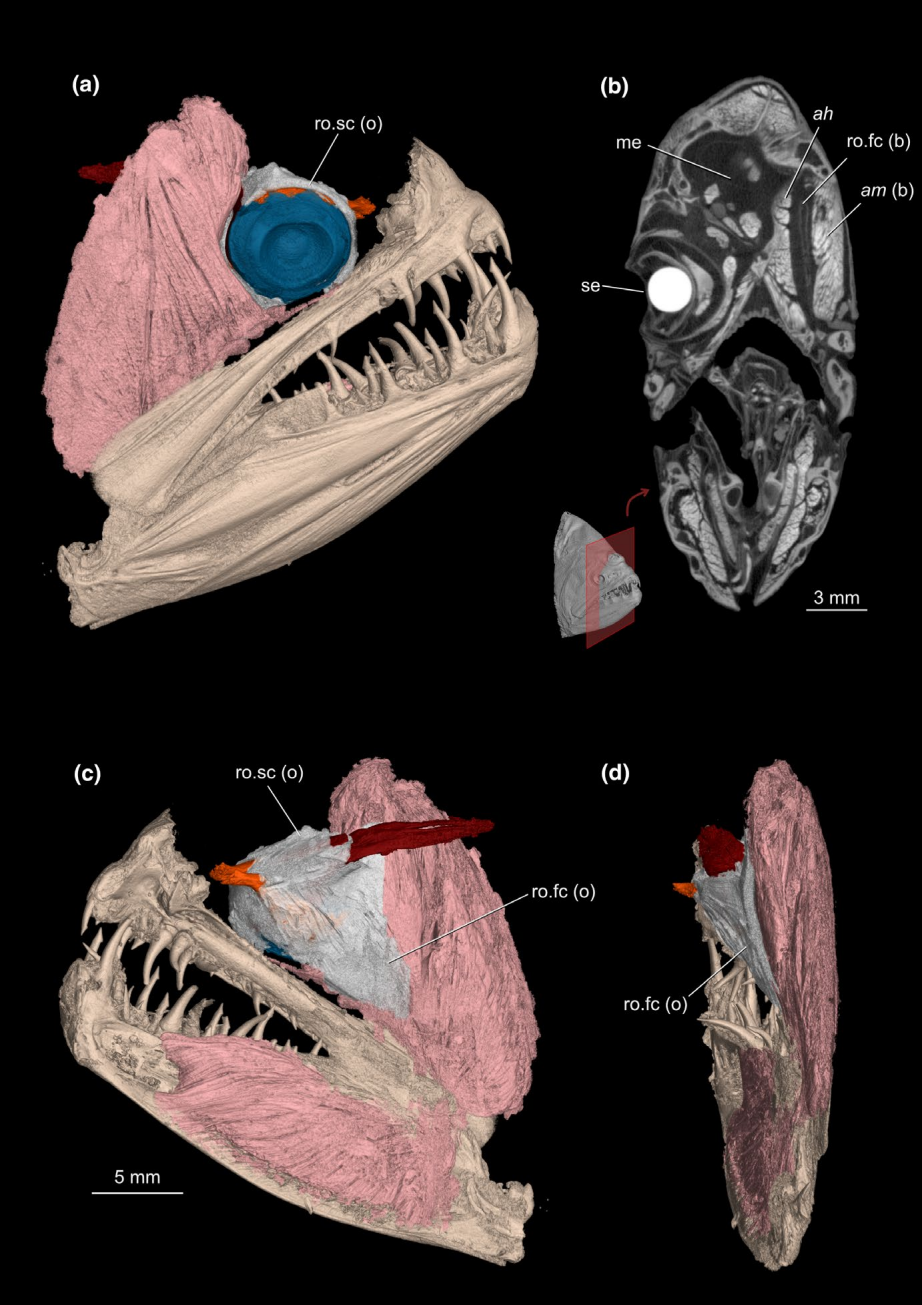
X‐ray microcomputed tomographies of the mandibular jaw of the ocular side of *Psettodes erumei* showing the facial segment of the *adductor mandibulae* and the sessile eye with its associated muscles and *recessus orbitalis*. (a) Lateral view, (b) transversal section of the head showing the position of the blind side *recessus orbitalis*, sandwiched in between the facial segment of the *adductor mandibulae* and *adductor hyomandibulae*, (c) medial view, (d) Posterior view. ah, *adductor hyomandibulae*; am, facial segment of the *adductor mandibulae*; (b) blind side; me, Migrated eye; (o) ocular side; ro.fc, *recessus orbitalis*, facial chamber; ro.sc, *recessus orbitalis*, scleral chamber; se, sessile eye.
